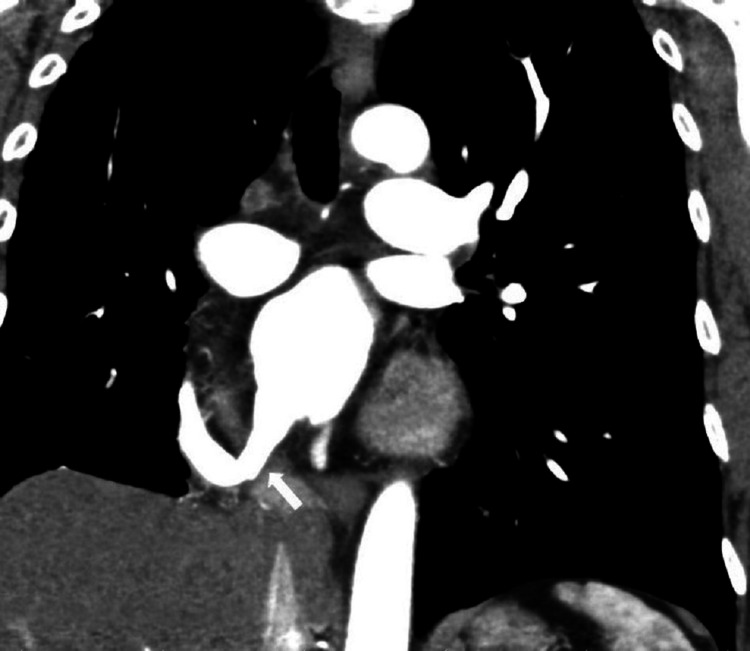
Post-intervention CT image. Solitary right pulmonary vein after dilatation (arrow).
